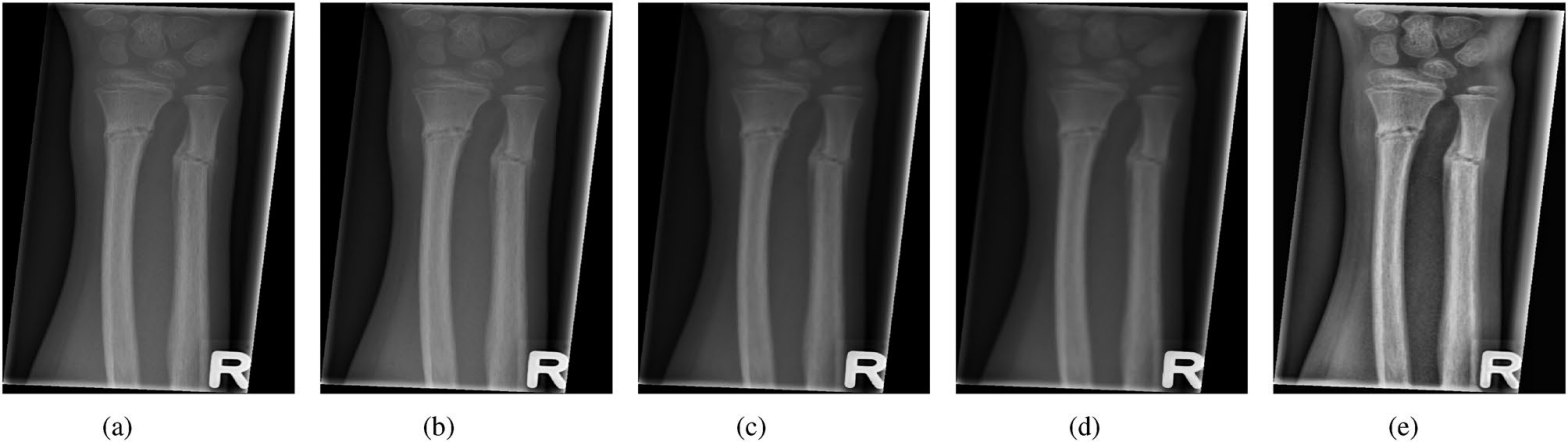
Image enhancement results applied to X-ray Images.
